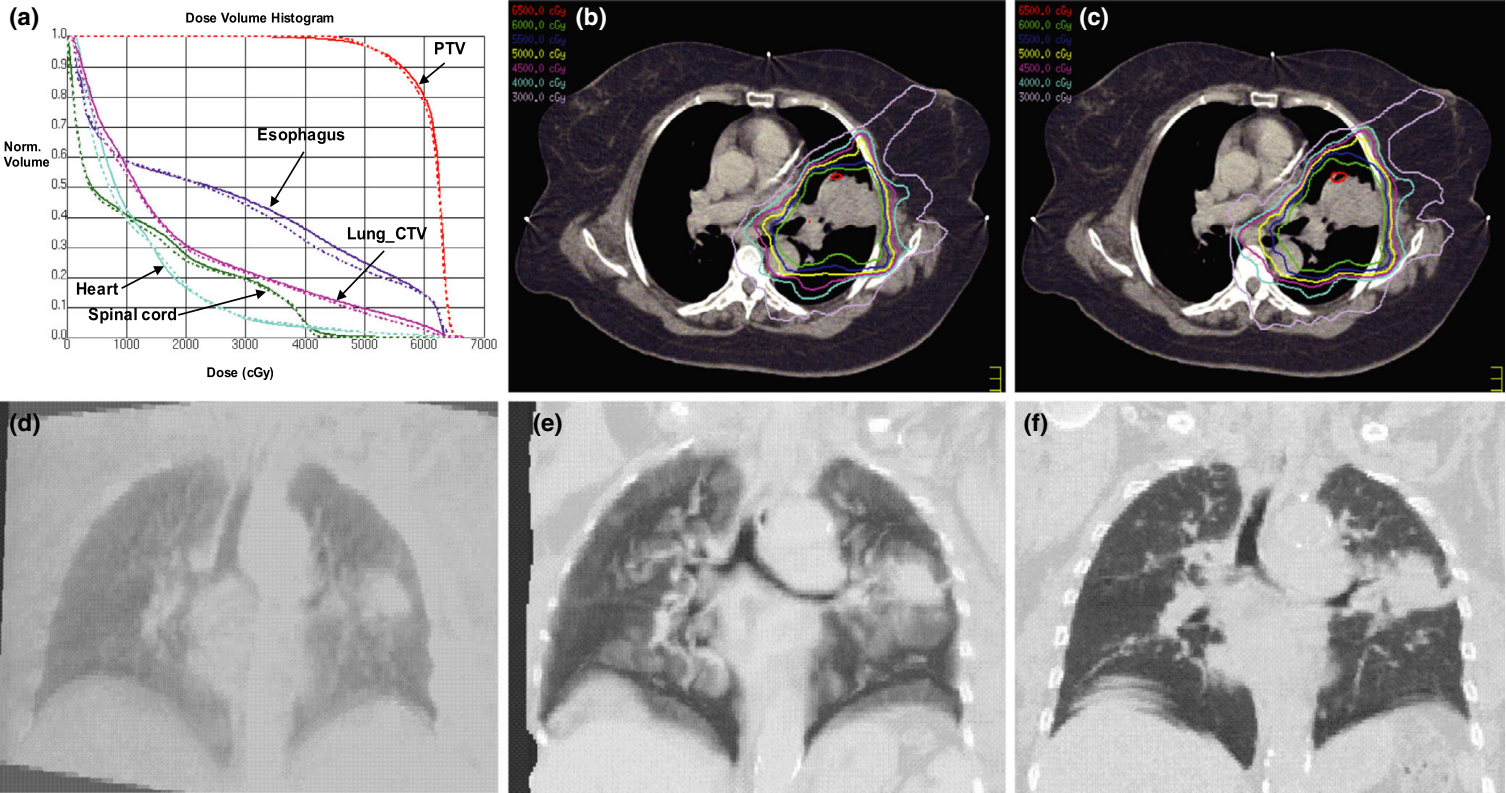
A case exhibiting poor agreement between vCT (dash line) and rCT (solid line) based accumulated doses (a), and isodose distribution comparison between vCT‐based accumulated dose (b) and rCT‐based accumulated dose (c), with their corresponding CBCT (d), vCT (e), and rCT (f).CBCT, cone beam CT.
